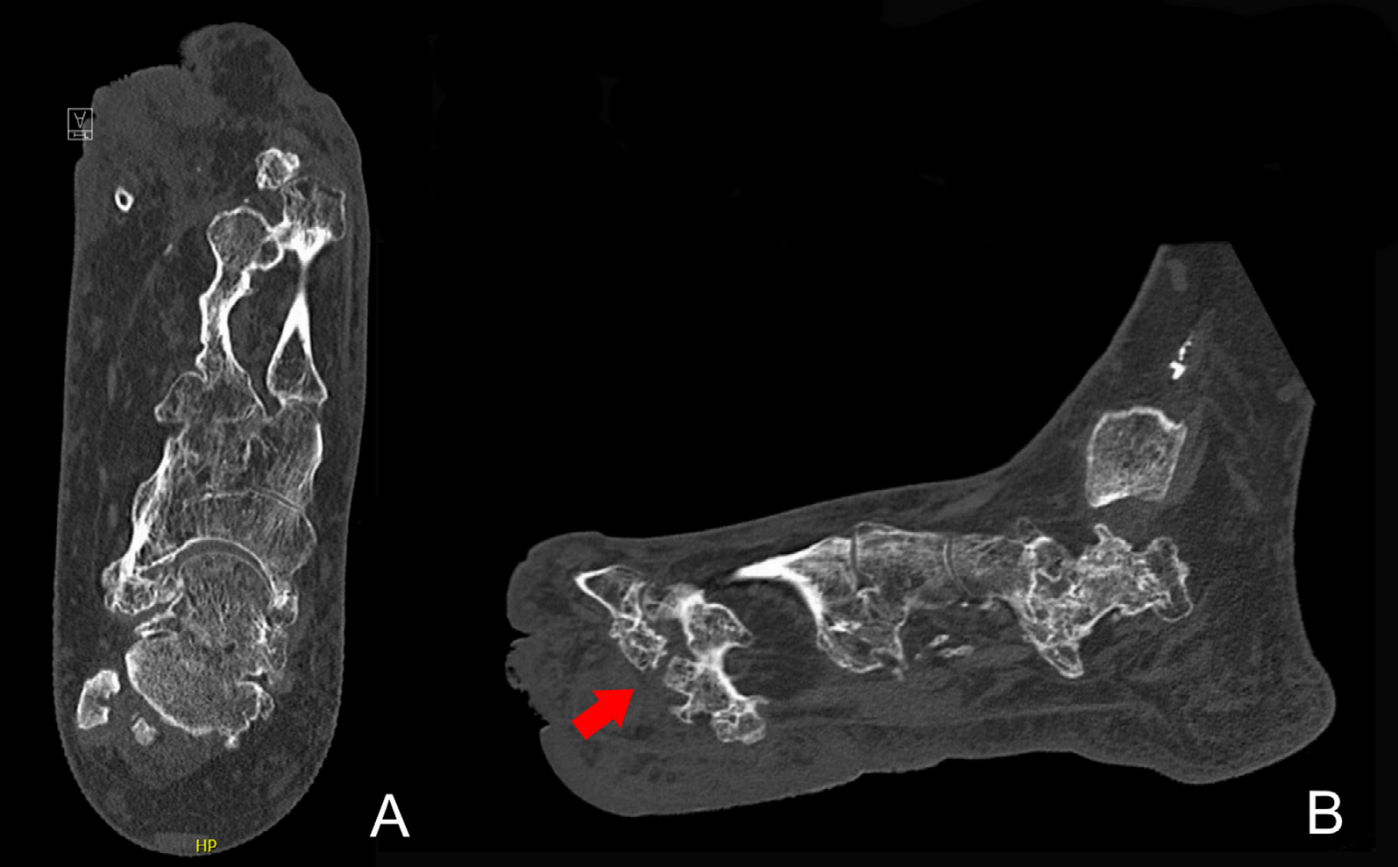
CT-scan of the left foot of case 1. Status after amputation of the first and second toe in 1962 and additional soft tissue debulking surgery in 1964, 1965, 1973, and 1978. 3A: Transversal view showing a synostosis between metatarsal I and II. 3B: Sagittal view with a visible bone spur from metatarsal II toward the plantar side (arrow).
